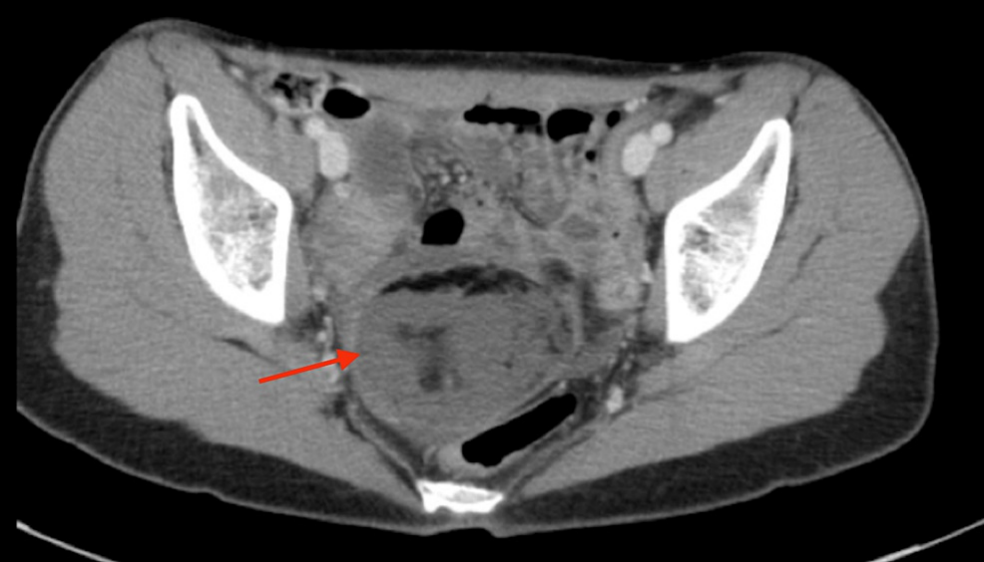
Axial view of the CT abdomen/pelvis showing the complex mass (red arrow). CT: computed tomography
